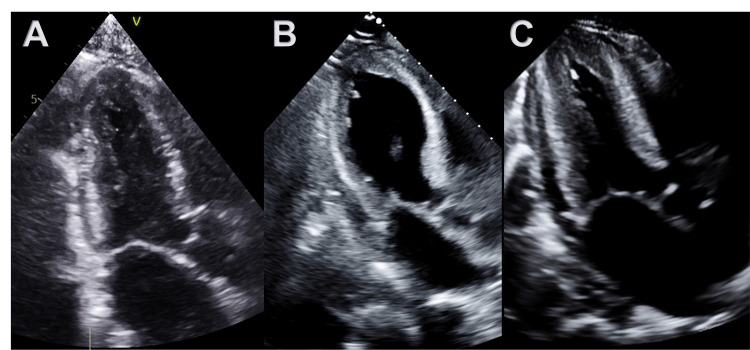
Echocardiography images (apical 3-chamber view) (A) Normal apical systole four months pre-op, (B) mid and distal anterior septum and entire apex are akinetic with preserved contraction in basal portion at six days post-OLT, (C) Recovered LV function with normal apical systole 20 days post-OLT OLT: orthotopic liver transplantation; LV: left ventricle
